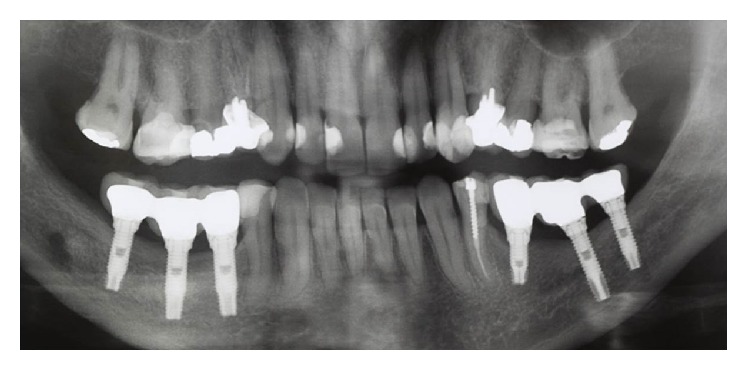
Panoramic X-rays after prosthetic rehabilitation.
